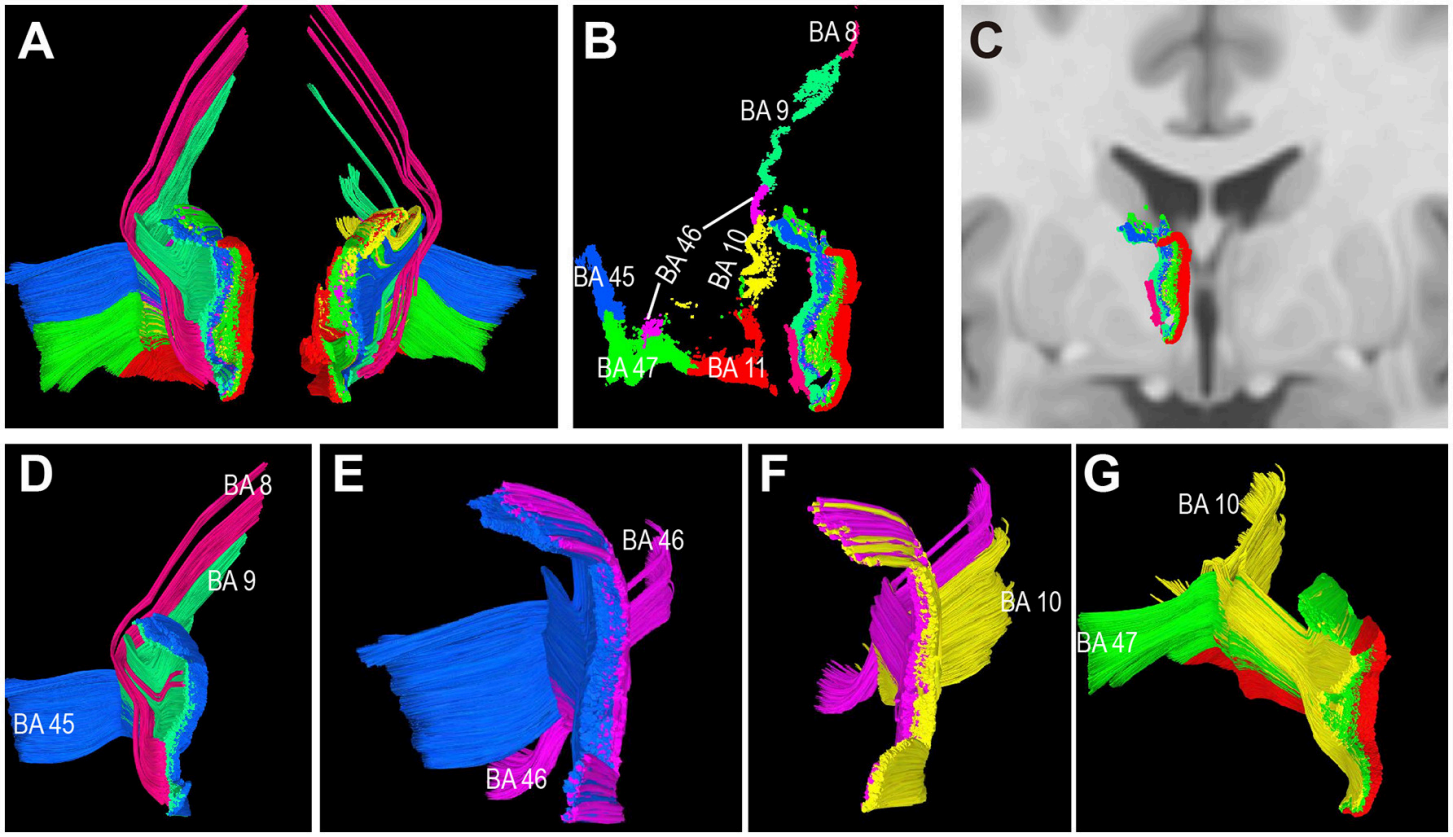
Connectivity patterns of the human thalamic-prefrontal peduncle (TPP) fibers (HCP-842 template). Posterior view: (A) Tracts of the left and right TPP. Fibers are assigned different colors based on the different prefrontal Brodmann cortical areas. (B) Endpoints of the left TPP show the connectivity patterns of the human TPP. TPP fibers connected the prefrontal cortex with the thalamus layer by layer. Subtracts terminate in the thalamus sequentially as follows: (from medial to lateral) BA 11, BA 47, BA10, BA 46, BA 45, BA9, and BA8. (C) The left TPP endpoints in the thalamus are overlaid on T1 coronal MRI slices. (D–G) Spatial relationship among subtracts. (D) BA 8 tract is located at the most lateral part of the TPP; th eBA 9 tract runs medially to the BA 8 tract; the BA 45 tract is located medial-ventrally to the BA9 tract. (E) The BA 46 tract runs medially to the BA 45 tract and splits into two parts (dorsal and ventral tracts). (F) The BA 10 tract runs medially to the BA 46 tract. (G) The BA 47 tract runs between the BA 11 and BA 10 tracts, then turns laterally to the cortex-crossing part of the BA 10 tract.
